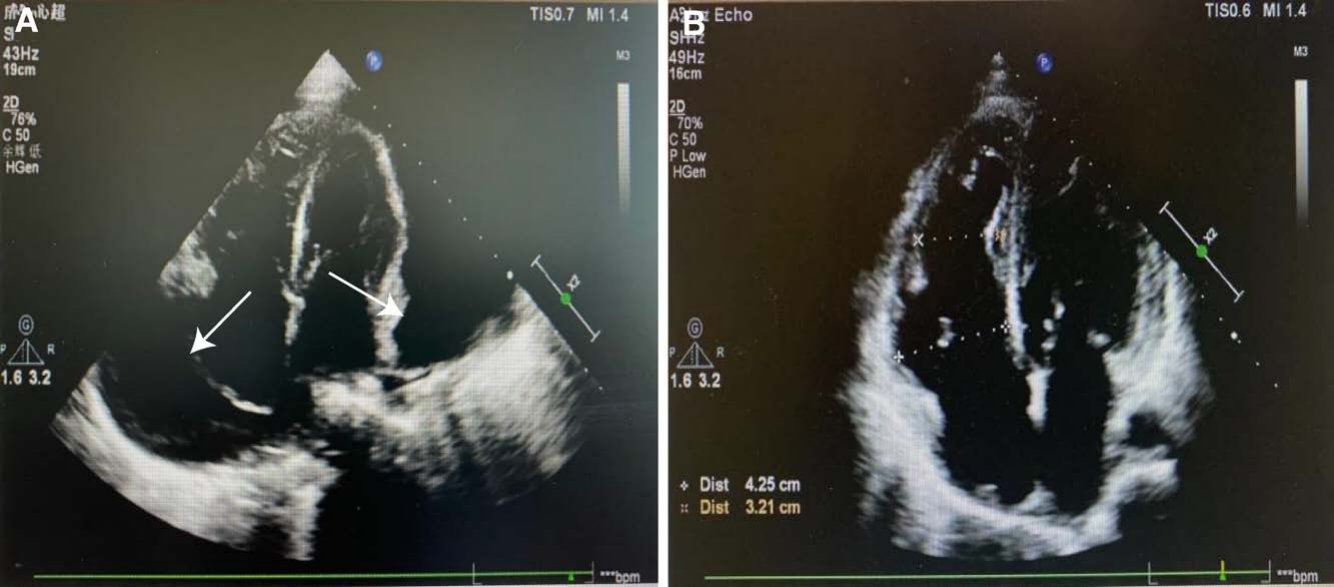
Echocardiography before and 5 mo after sintilimab administration. (A) An initial echocardiography revealed massive pericardial effusion (white arrow). (B) A follow-up echocardiography 5 mo after sintilimab administration showed that the patient’s pericardial effusion was completely absorbed.
